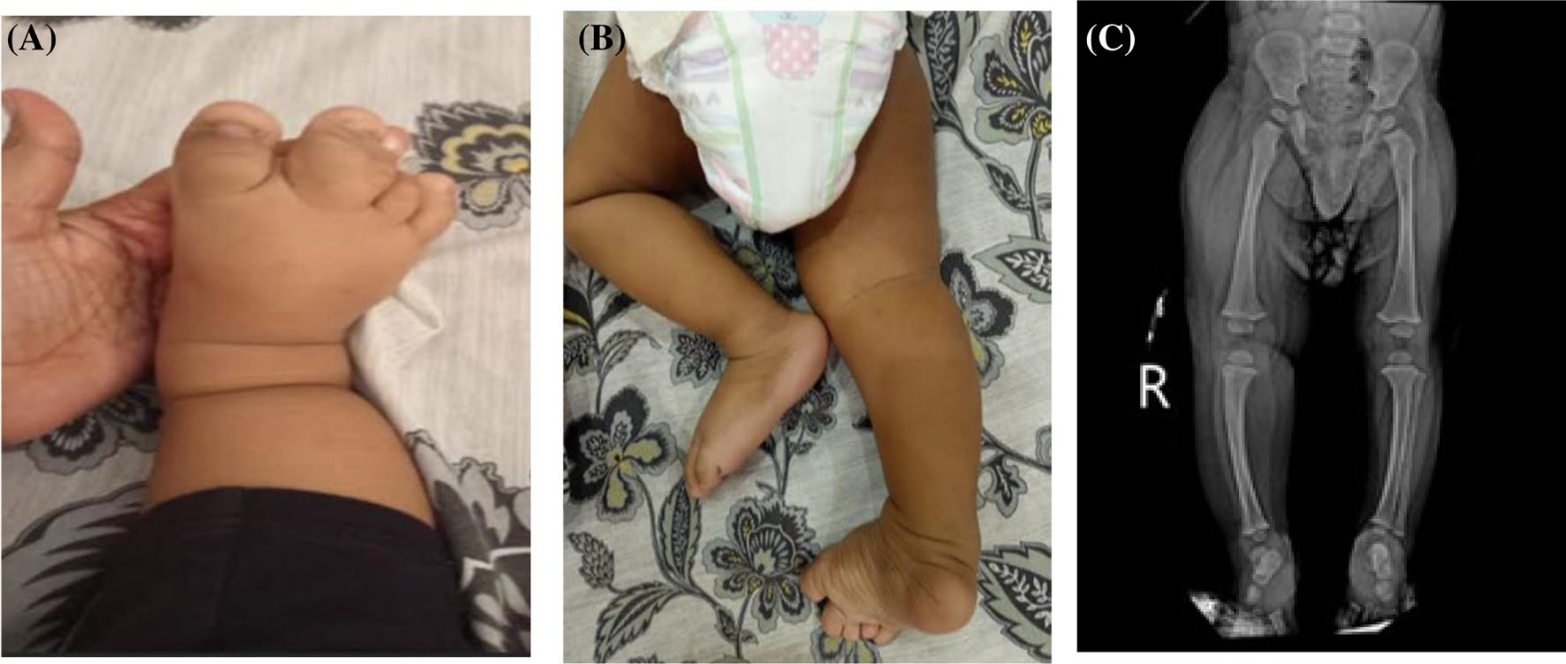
(A) Syndactyly of the right foot accompanied by localized gigantism of the right lower limb. (B) Prone‐position view demonstrating increased circumference of the right leg compared with the left. (C) Anteroposterior radiograph of the lower limbs showing increased soft‐tissue thickness on the right side, with no evidence of bony hypertrophy.
